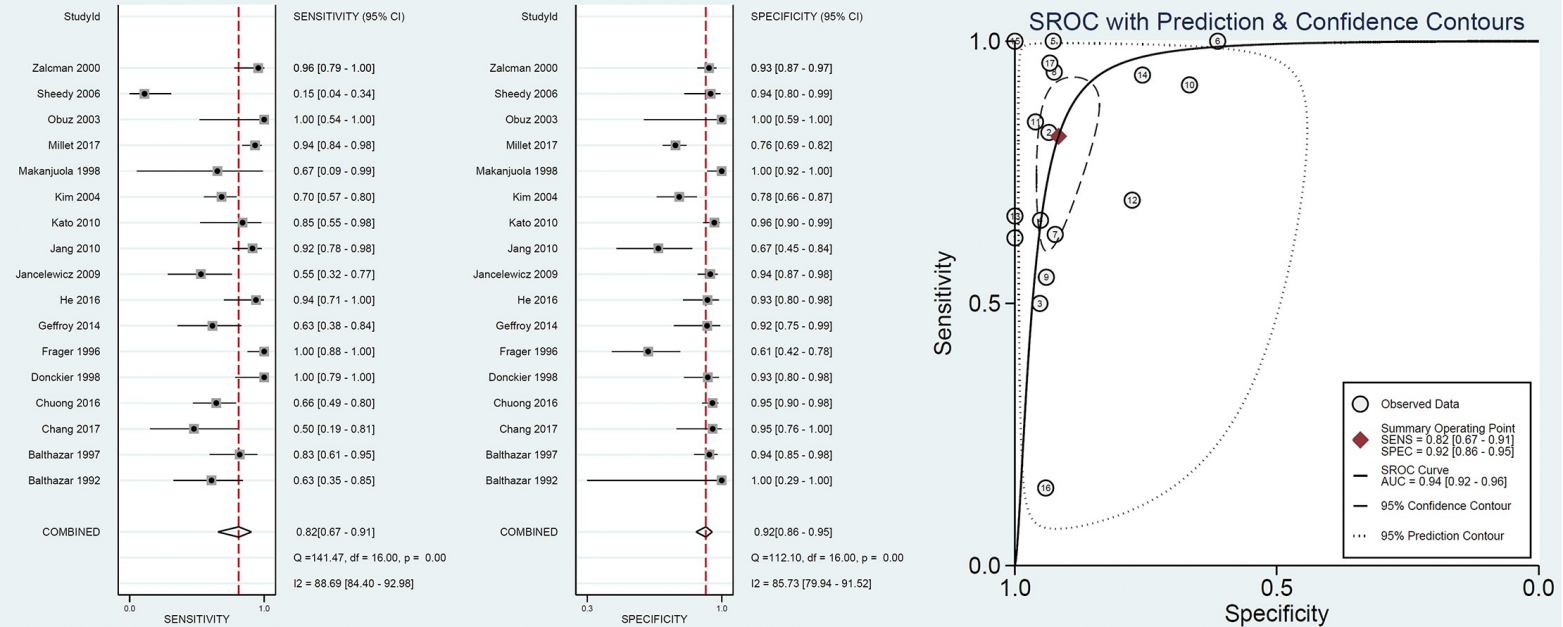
Value of CT in diagnosis of ischemia.
